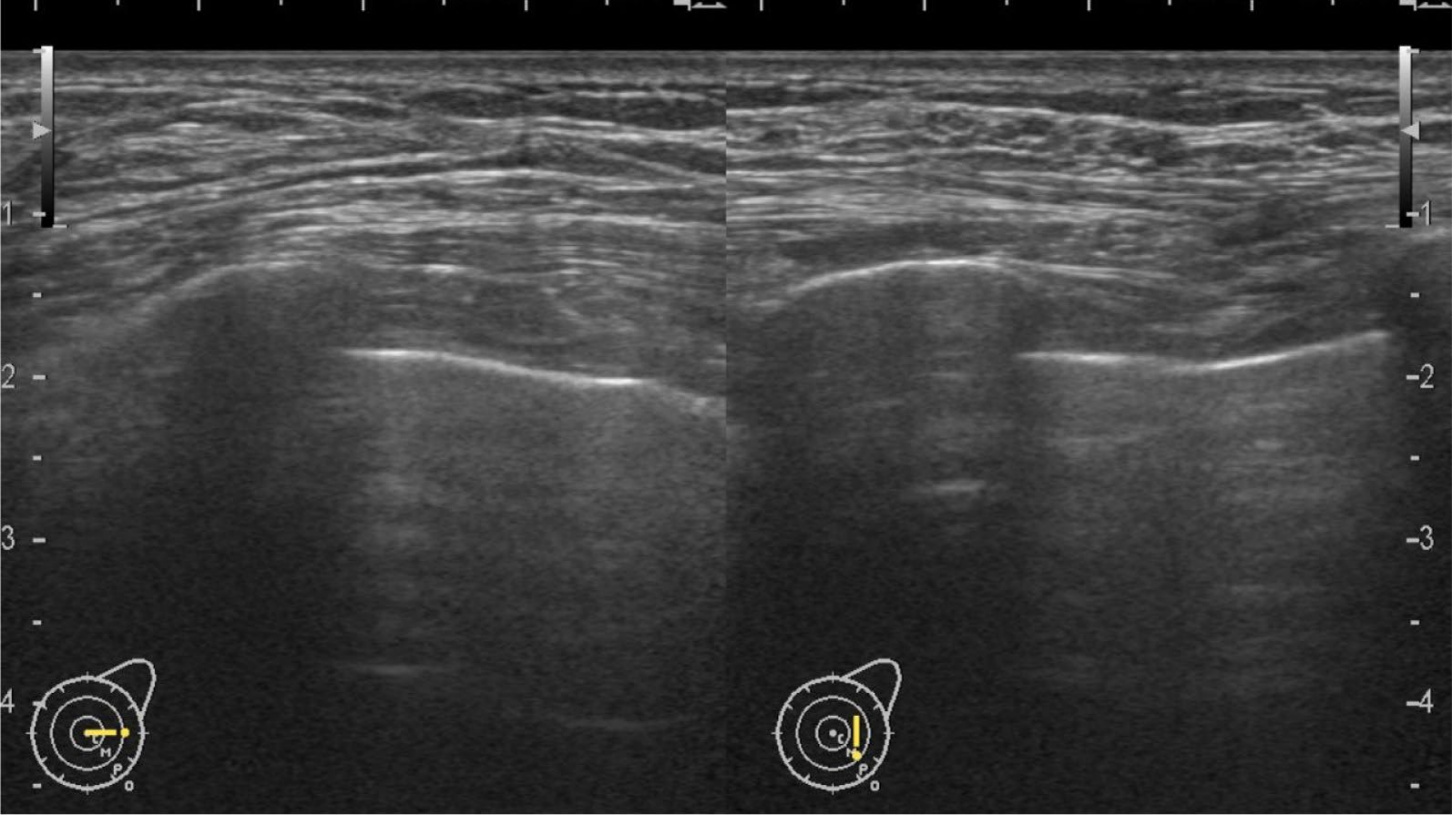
A month after the patient’s first visit, we could not detect the tumor on ultrasonography
